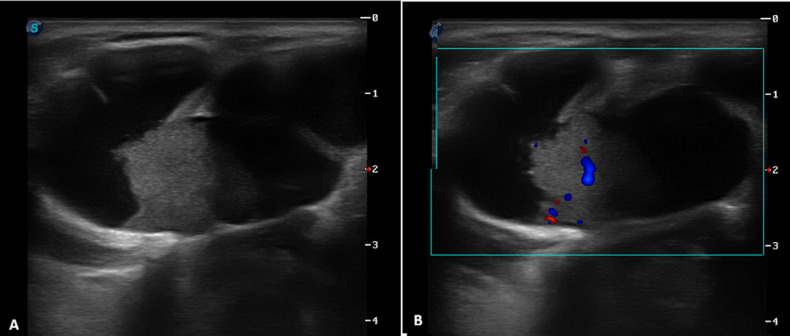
cross-section of cervical ultrasound showing a septated midline cyst located above the thyroid cartilage, measuring 28x50x30 mm; B) an isoechoic tissue component adherent to the posterior cyst wall, measuring 12x11x10 mm, with central vascularization
